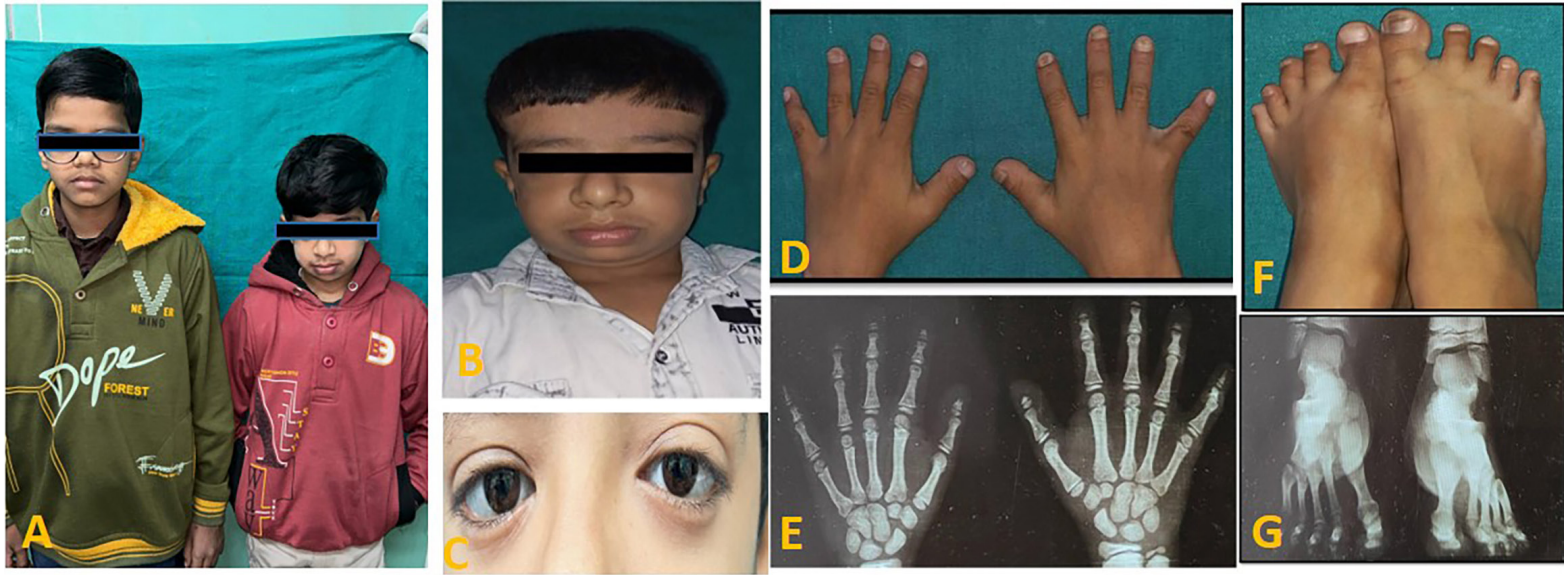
A to G: (A) Non-identical twins in which one is affected and the other is normal (B) Extraoral view. (C) Bluish sclera and hypertelorism (D) Short, stubby fingers-Brachydactyly (E)Antero-posterior radiograph of the hands showing acro osteolysis (F)Short feet with distal phalanges short (G)Antero-posterior radiograph of the feet showing sandal gap deformity and acro osteolysis.
